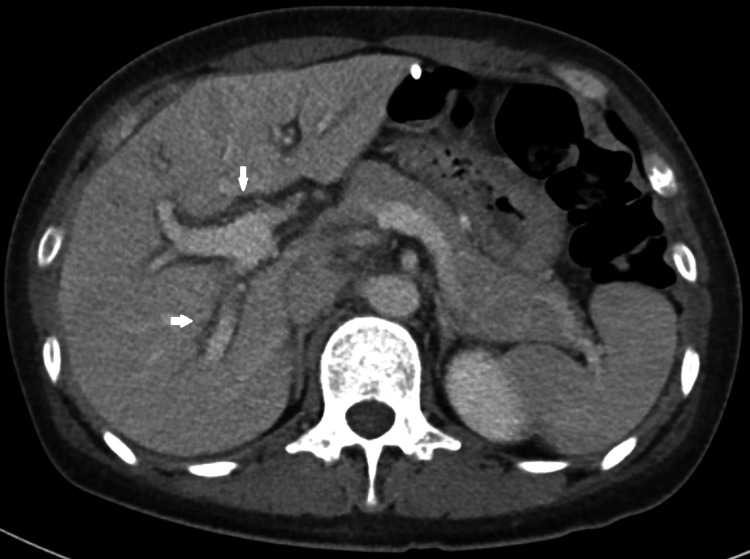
CT Abdomen/Pelvis with IV contrast (Axial view) A CT scan of the abdomen and pelvis obtained on admission showing mild hepatomegaly, diffuse irregularity of the hepatic contour, and periportal edema (white arrows).
